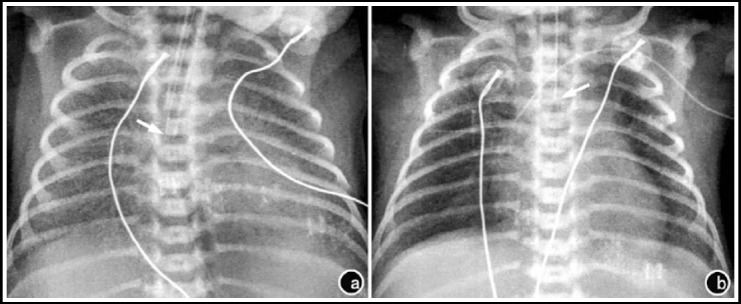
Pulmonary X-ray results of neonate No. 2 before and after treatment. a) 50 minutes after birth, before treatment; b) 8 hour after PS treatment.
